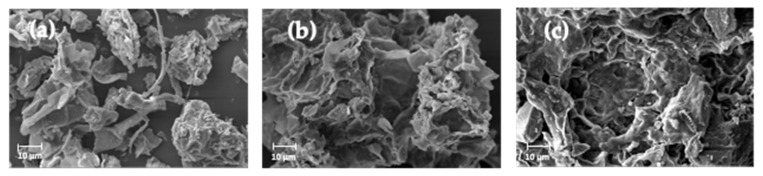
SEM images for (a) duckweed flour, (b) control protein extract, and (c) ultrasound protein extract at a magnification of 1000×.
